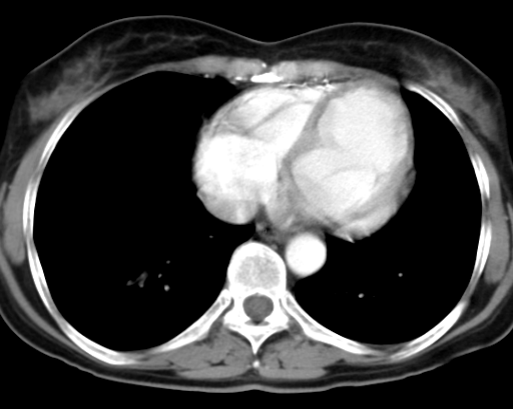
Thoracic CT scan after the tumor was excized. Normal heart. No pericardial free space in this section plan.
